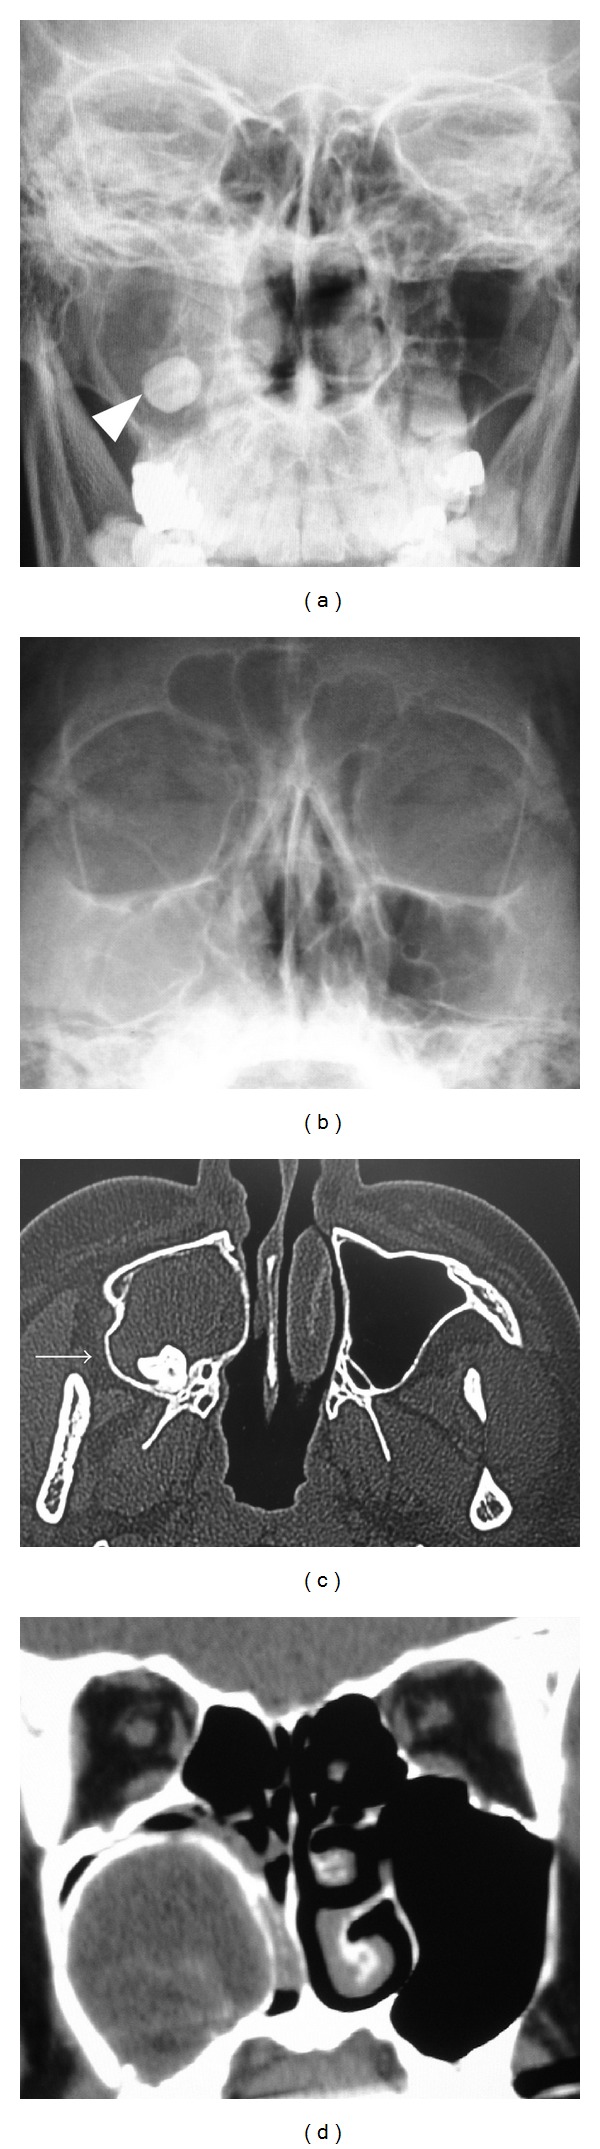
(a) X-ray shows cloudy appearance in the right maxillary sinus including an impacted tooth (arrowhead) in the Caldwell view. (b) X-ray shows cloudy appearance in the right maxillary sinus in the Waters view. (c) Axial view of the CT scan shows a cystic lesion expanding the lateral wall (arrow) of the maxillary sinus containing the impacted tooth inside. (d) Coronal view of the CT scan shows the calcificated cystic wall.
